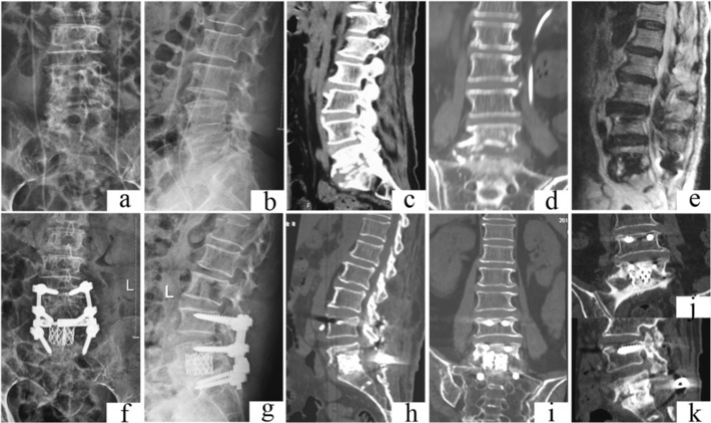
Case number 11. Preoperative image evaluation. a, b Radiograph showing L5–S1 involvement. c–d Computed tomography (CT) and e magnetic resonance imagery (MRI). f–j Postoperative radiography and h–i CT. j, k At final follow-up, the internal fixation was in good shape and interbody fusion had been obtained, without signs of tuberculosis recurrence
